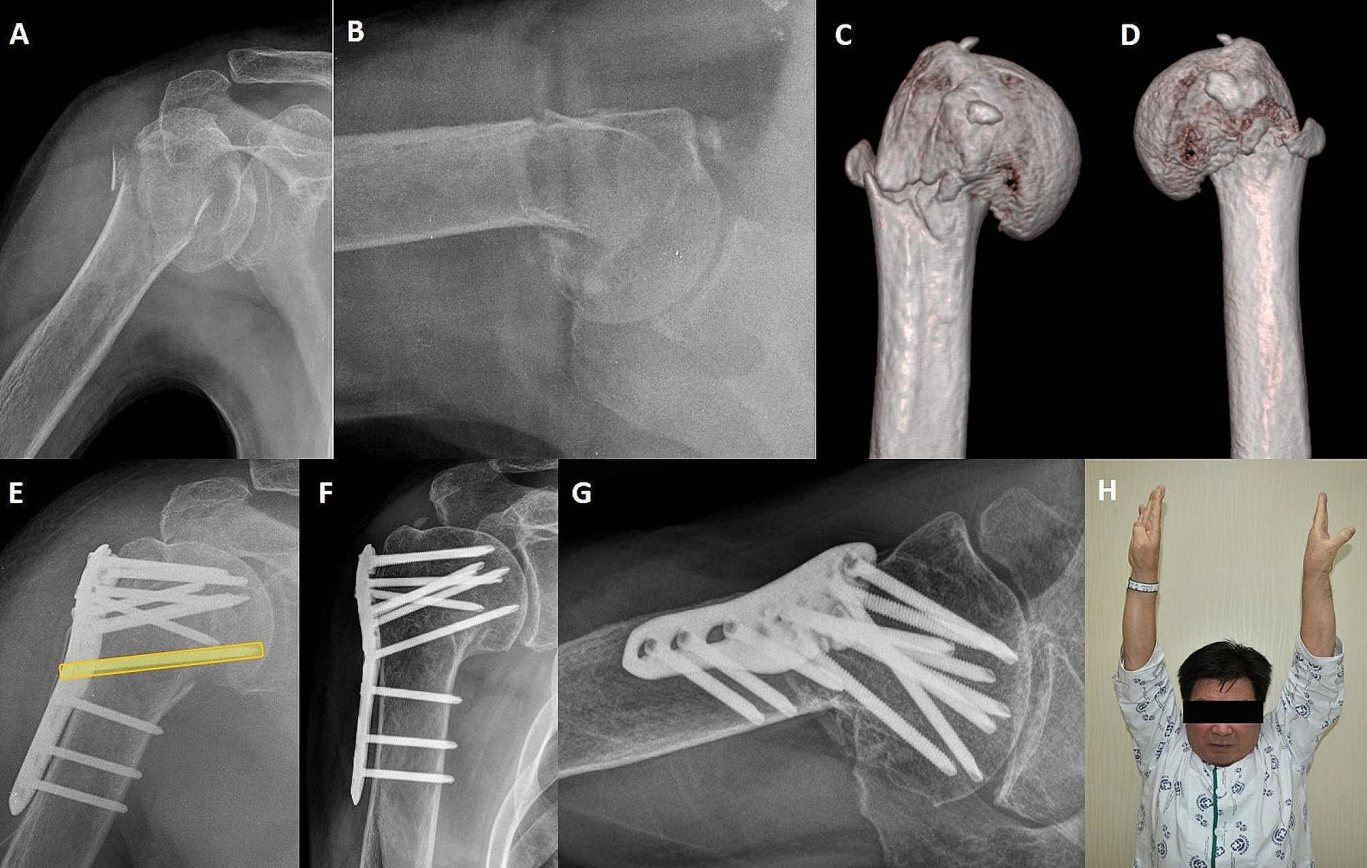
A case demonstrating favorable clinical and radiographic outcomes with a single medial supporting screw (MSS). A 71-year-old male patient sustained a right shoulder injury from a fall. The shoulder plain radiograph shows a right proximal humerus fracture (PHF) classified as a two-part fracture according to Neer’s classification in the (A) shoulder AP and (B) trans-axillary views. The (C) anterior and (D) posterior views of the 3D CT images reveal varus angulation of the proximal humerus accompanied by medial comminution. The (E) immediate postoperative radiograph shows the insertion of a single MSS. At 1 year postoperatively, (F) shoulder AP and (G) trans-axillary views show complete bony union at the fracture site without complications. (H) The patient exhibits a good range of motion comparable to the unaffected side at 1 year postoperatively
